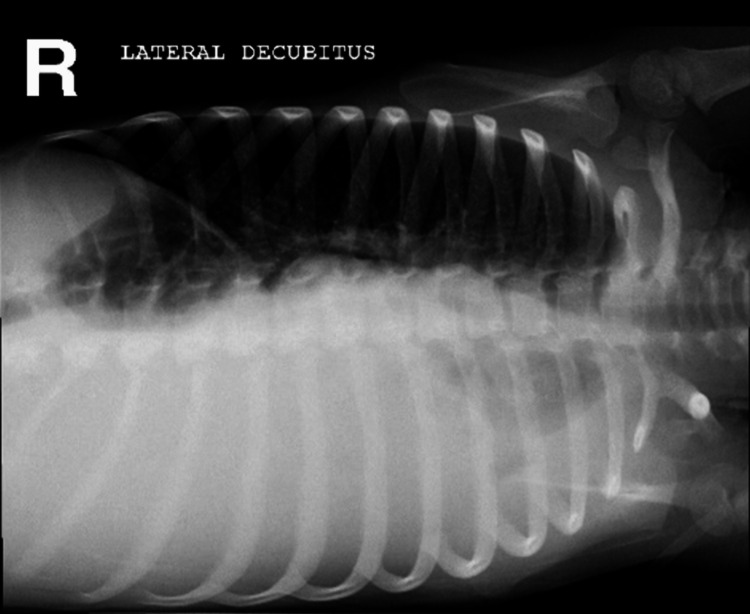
Plain chest X-ray, right lateral decubitus view, showing a large free fluid level occupying more than 50% of the ipsilateral hemithorax.
